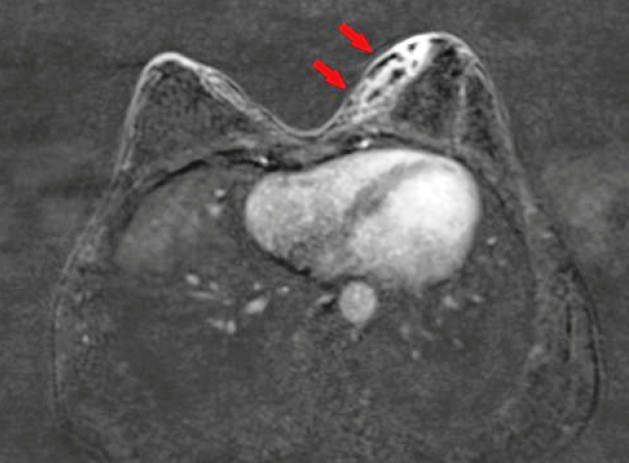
Axial T1 weighted MRI image: Pre-treatment image shows fistulized thick-walled abscess formation with thickening of the skin in the lower inner quadrant of the left breast (arrows).
